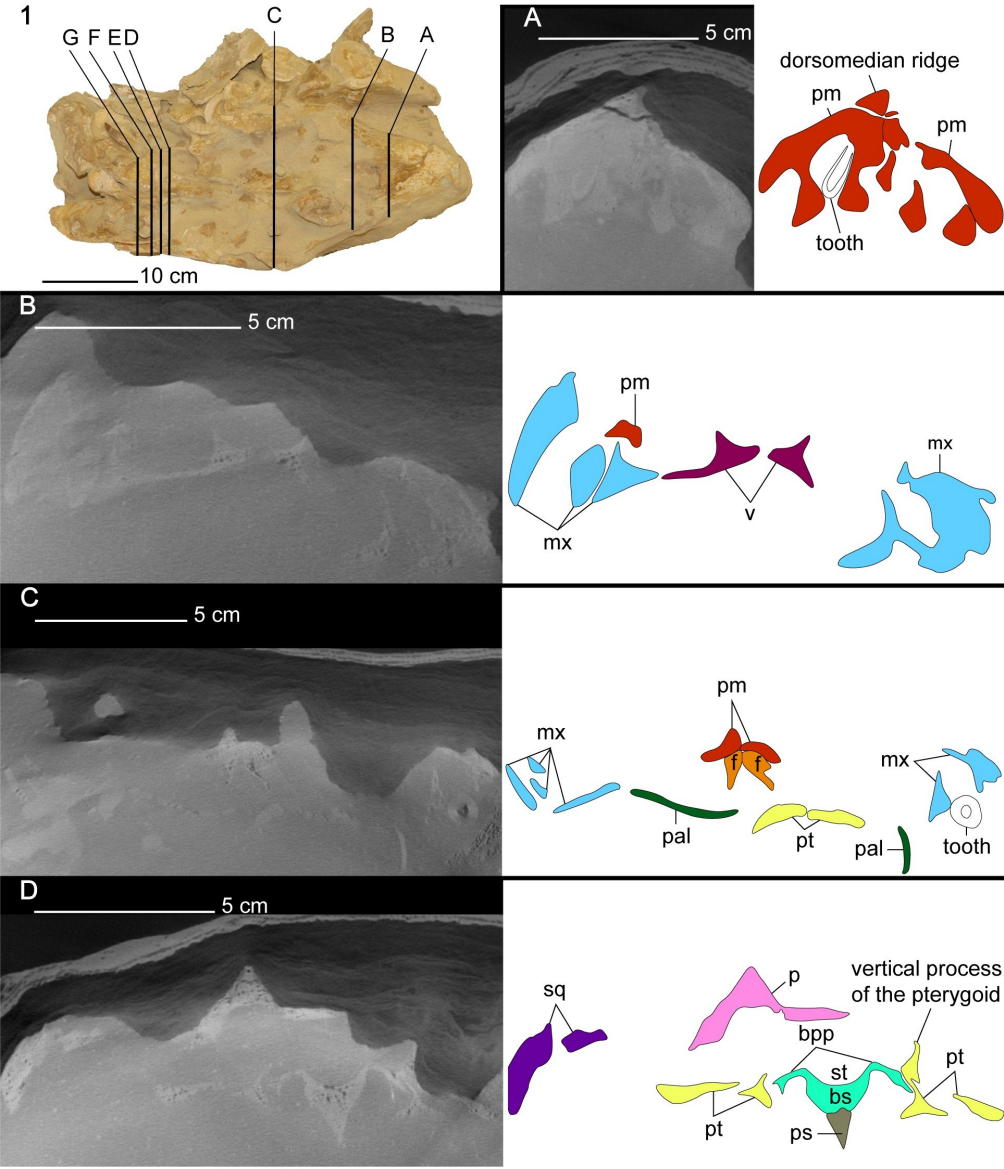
CT cross-section images of the cranium of *Cardiocorax mukulu* (MGUAN PA278). A. Transversal section through the third pair of premaxillary alveoli. B. anterior margin of the palate. C. Section of intraorbital region of the cranium. D. Section through the anterior margin of the sella turcica.
